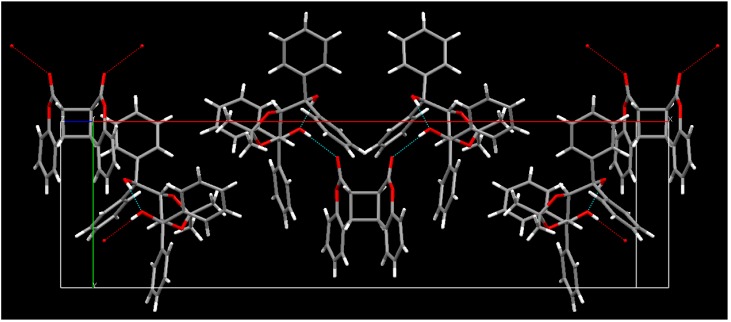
X-ray structure of the 2:1 complex of (−)-23 and the optically active *anti-head-head* dimer (−)-2.
